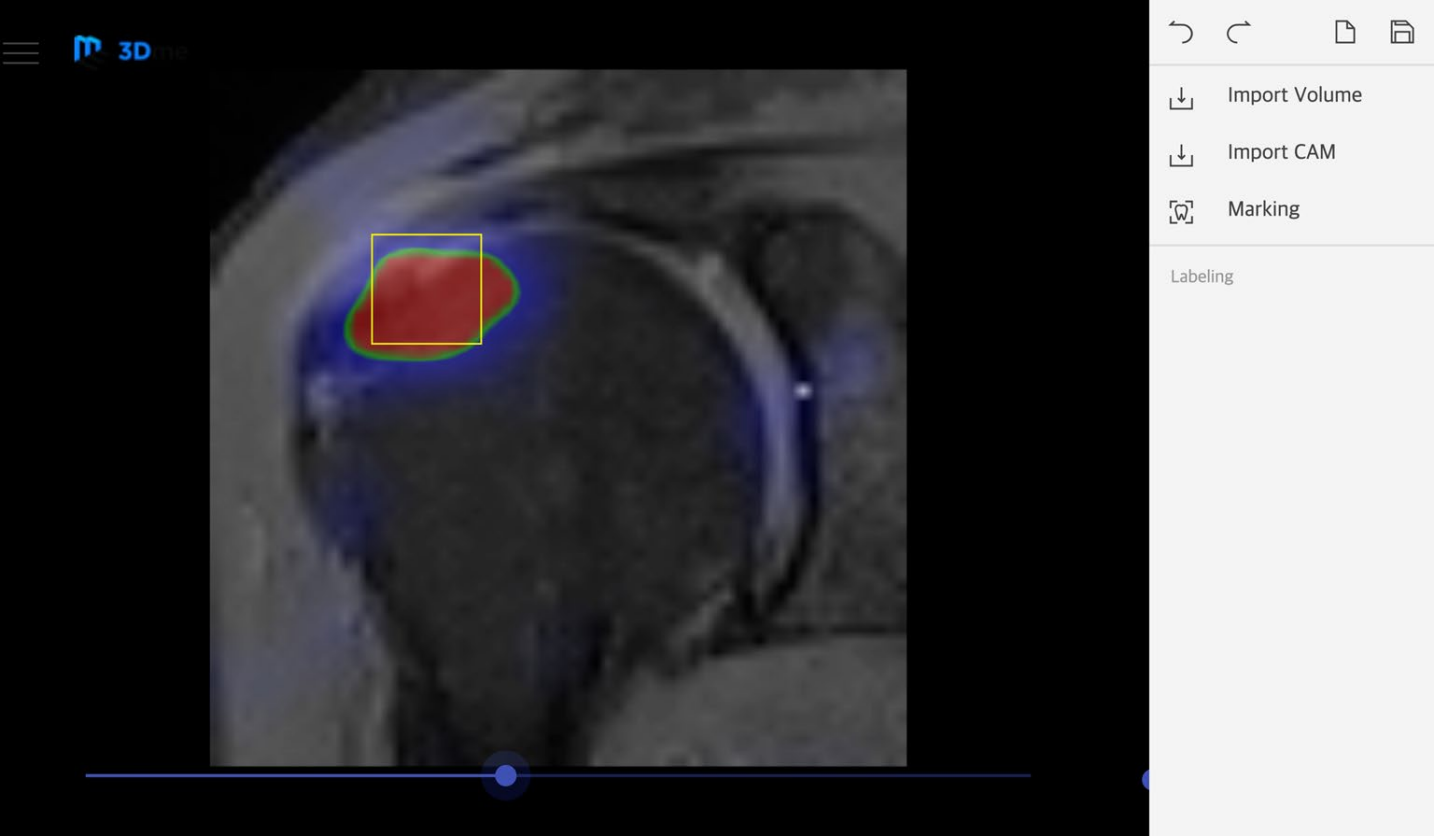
An example which shows the visualized CAM location is on the same position of RCT where an orthopedic shoulder specialist marked on the original MR image with a yellow box.
